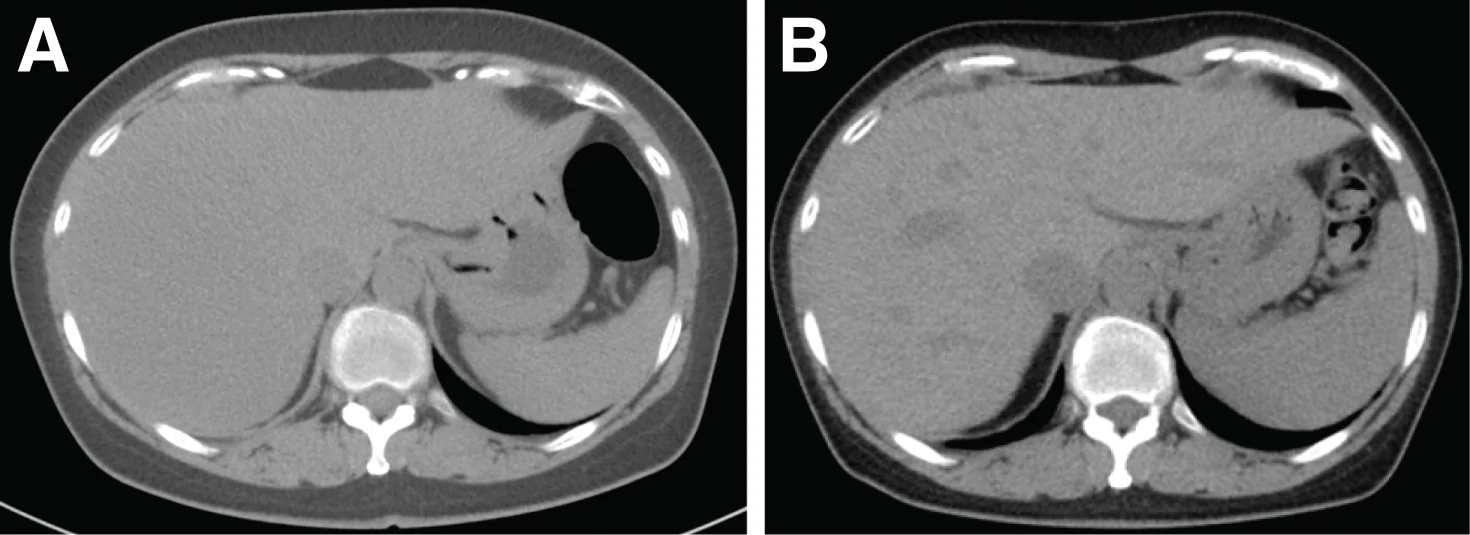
Non-contrast CT images before and after T-DM1 therapy. (A) Before initiation of T-DM1 therapy, the spleen measured 95.6 × 35.1 mm. (B) Two months after completion of T-DM1 therapy, the spleen had enlarged to 111.9 × 46.1 mm, consistent with splenomegaly. T-DM1, trastuzumab emtansine
